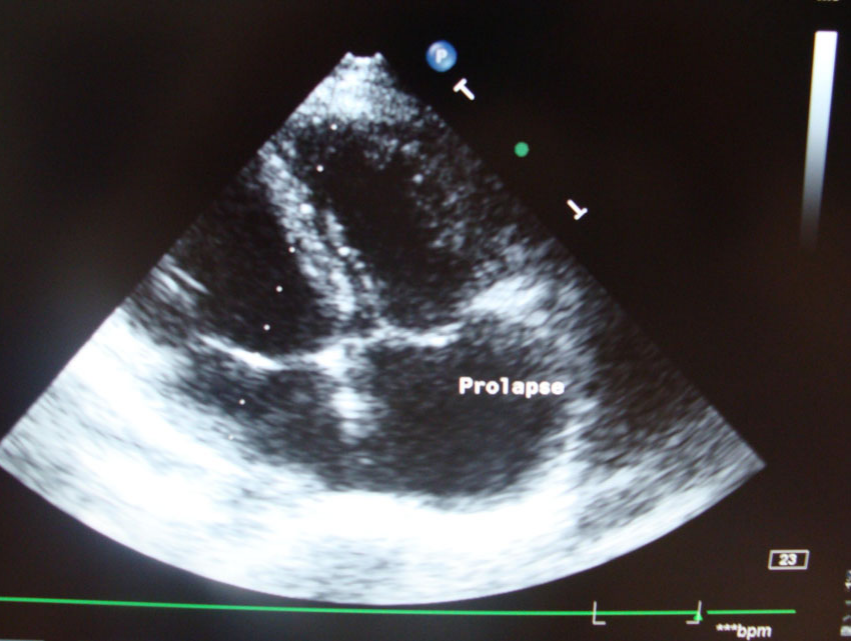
Thickend tricuspid valved, with its important prolapse, in apical four-chamber view of transthoracic echocardiography.
